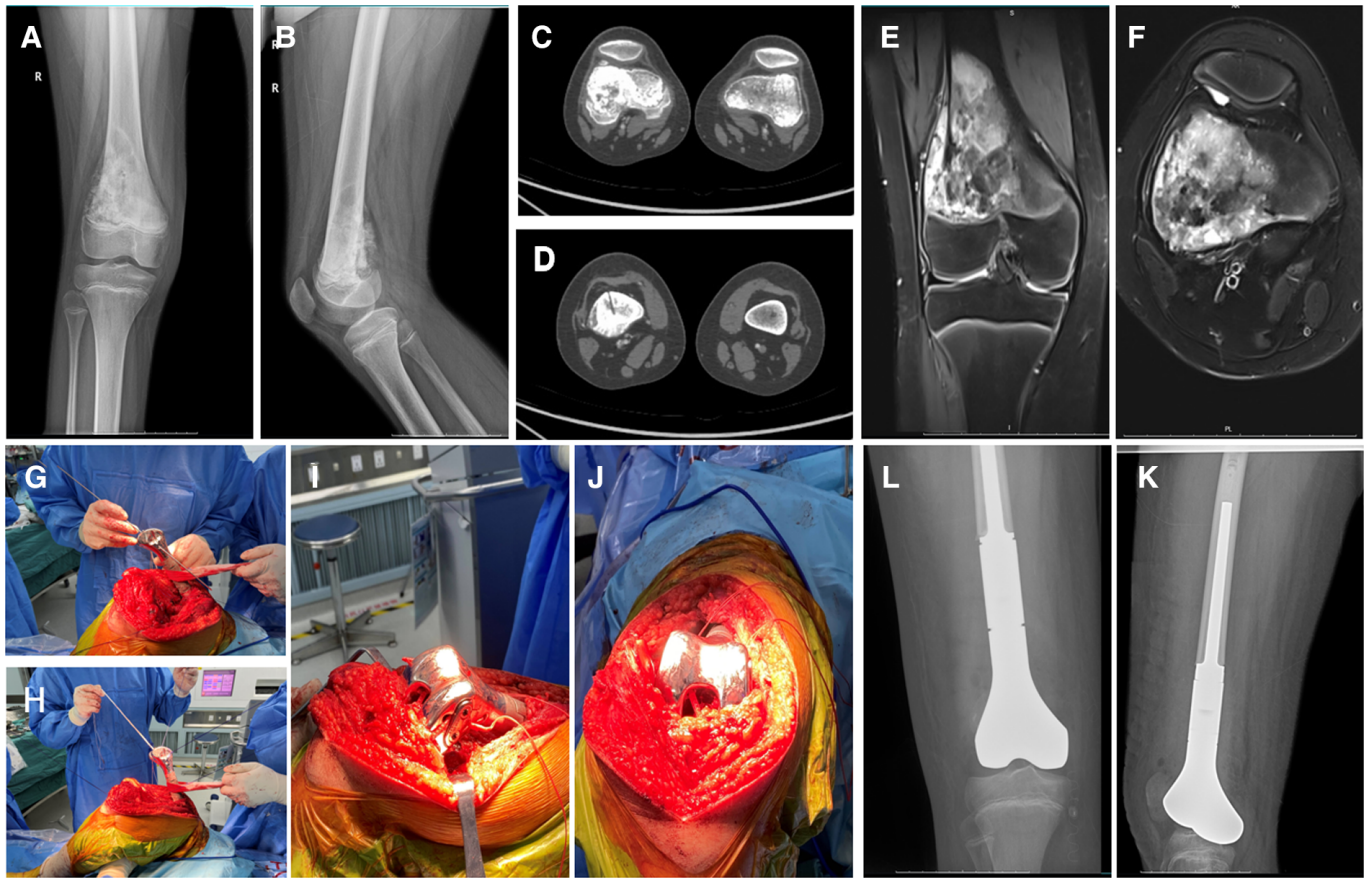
12 years old, male, osteosarcoma of the right distal femur, semi-joint prosthesis replacement combined ligament advanced reinforcement system (LARS) ligament reconstruction. (A,B) The radiograph of an osteosarcoma involving the metaphyseal region of the distal femur; (C,D) Preoperative CT indicated Osteoblastic destruction of the distal femur; (E,F) Preoperative MRI indicatedan abnormal signal area at the distal end of the right femur with a lateral soft tissue mass and tumor invasion of the epiphyseal line; (G,H) Methods of intraoperative LARS ligament reconstruction; (I,J) Appearance after LARS ligament reconstruction; (K,L) Postoperative x-ray of femur indicated that the prosthesis was in good position.
